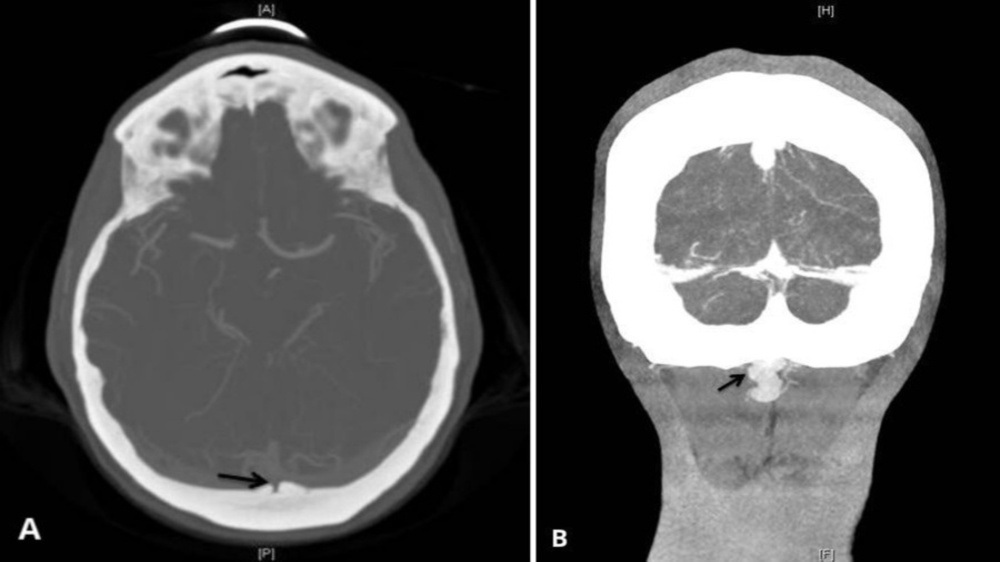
Axial (A), Coronal (B) views of CTA showing the penetration site of the central occipital emissary vein (arrows) to the cranium.
